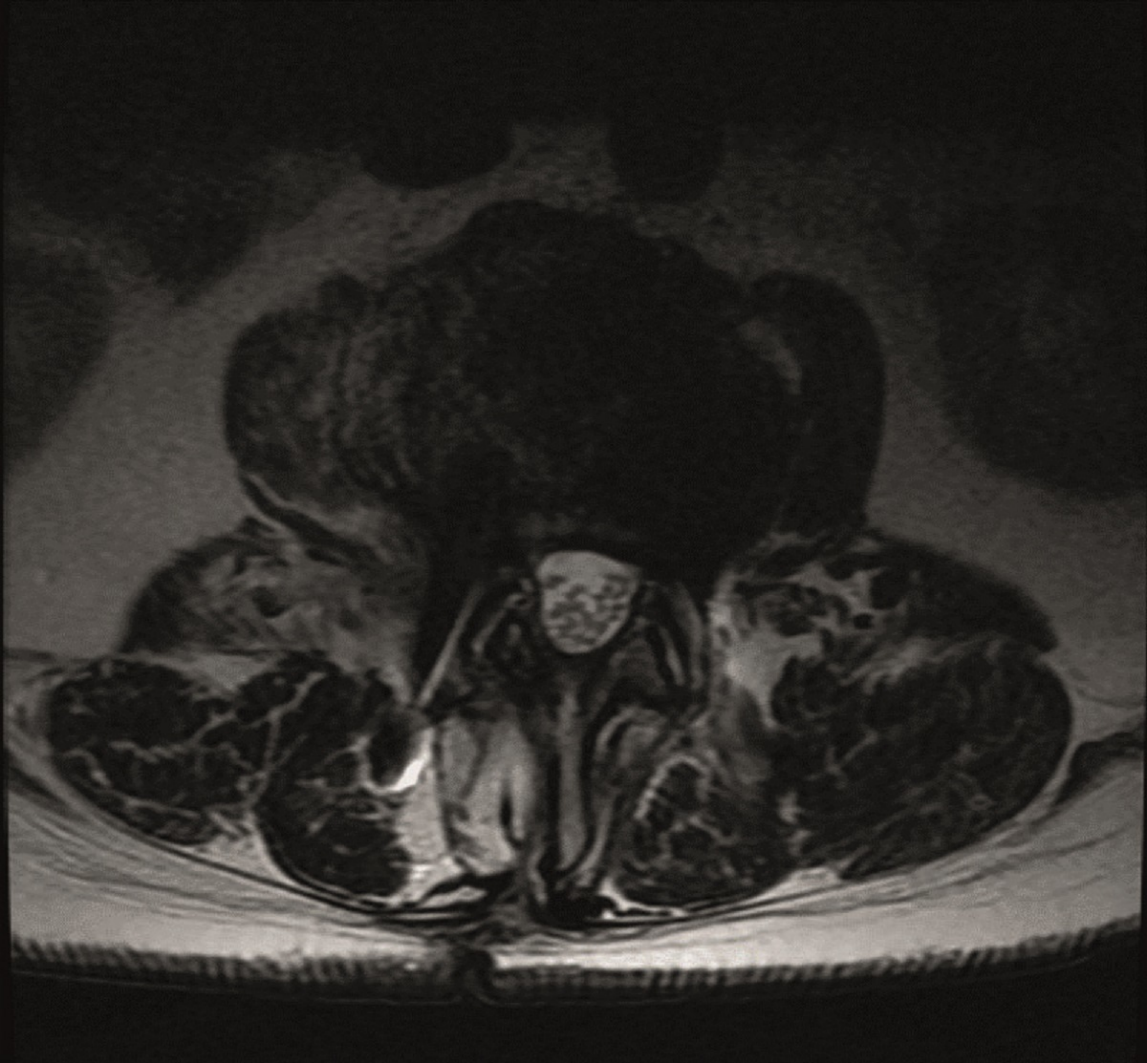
Axial T2 Post-operative MRI Interval marked widening of the spinal canal at L2-L3, with removal of the ligamentum flavum and the associated cyst.
